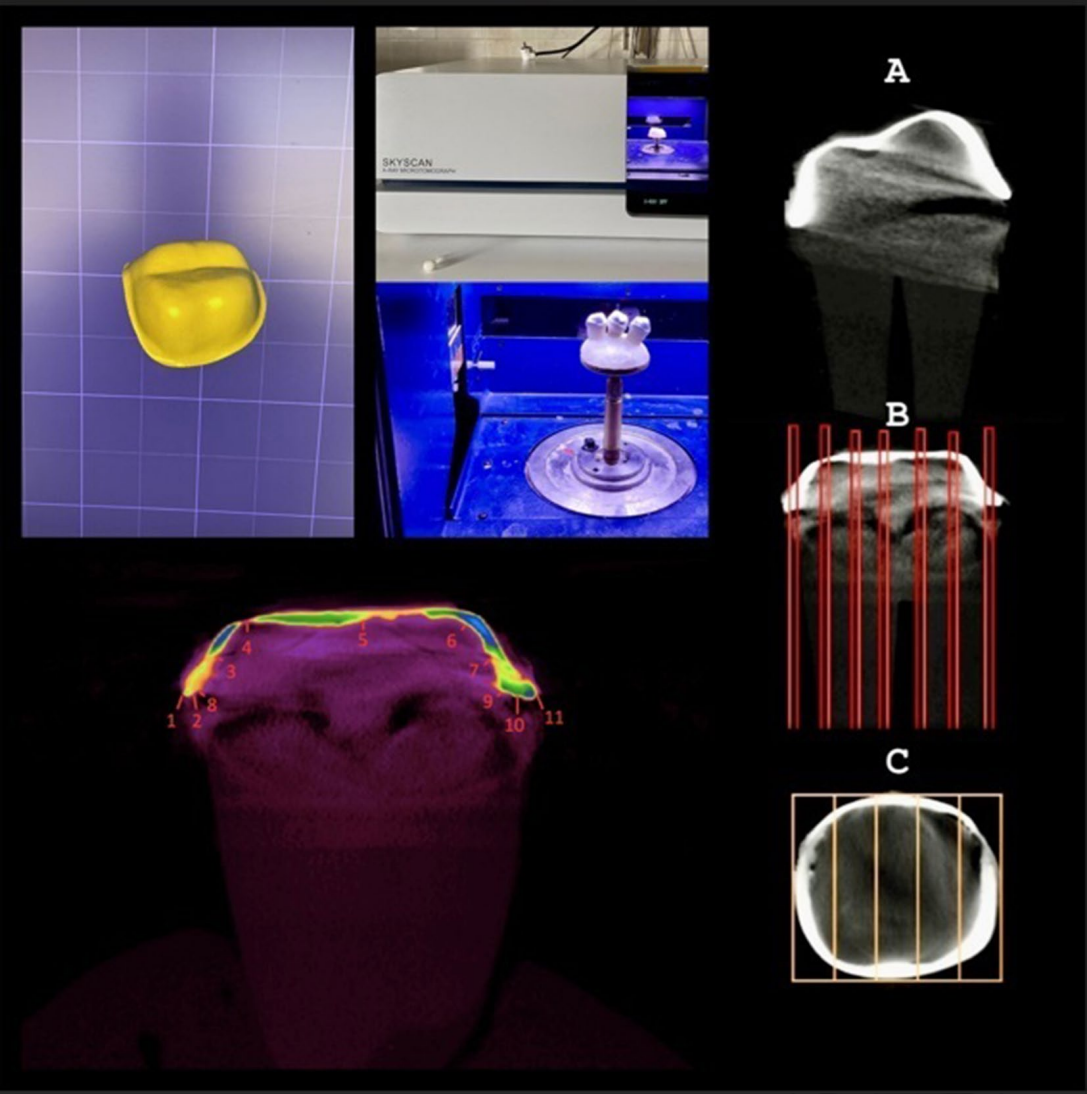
Representative of 2D and 3D micro CT measurements
